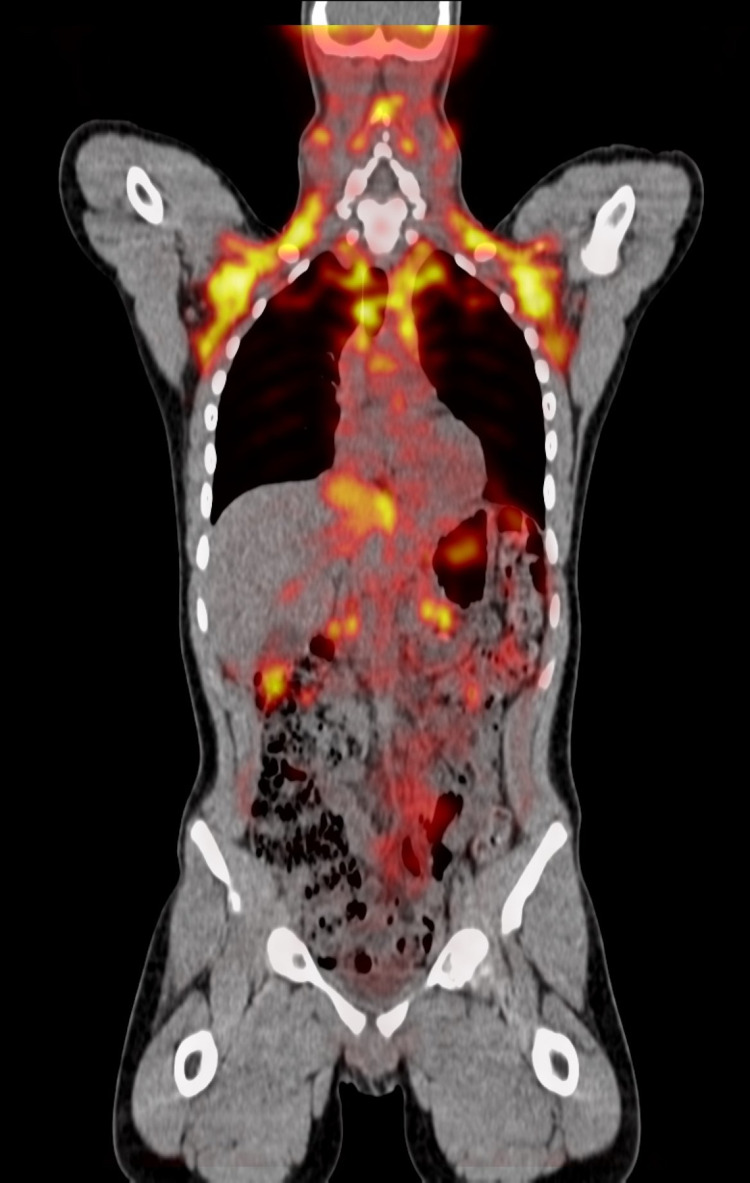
PET/CT did not reveal metastatic lesions.
